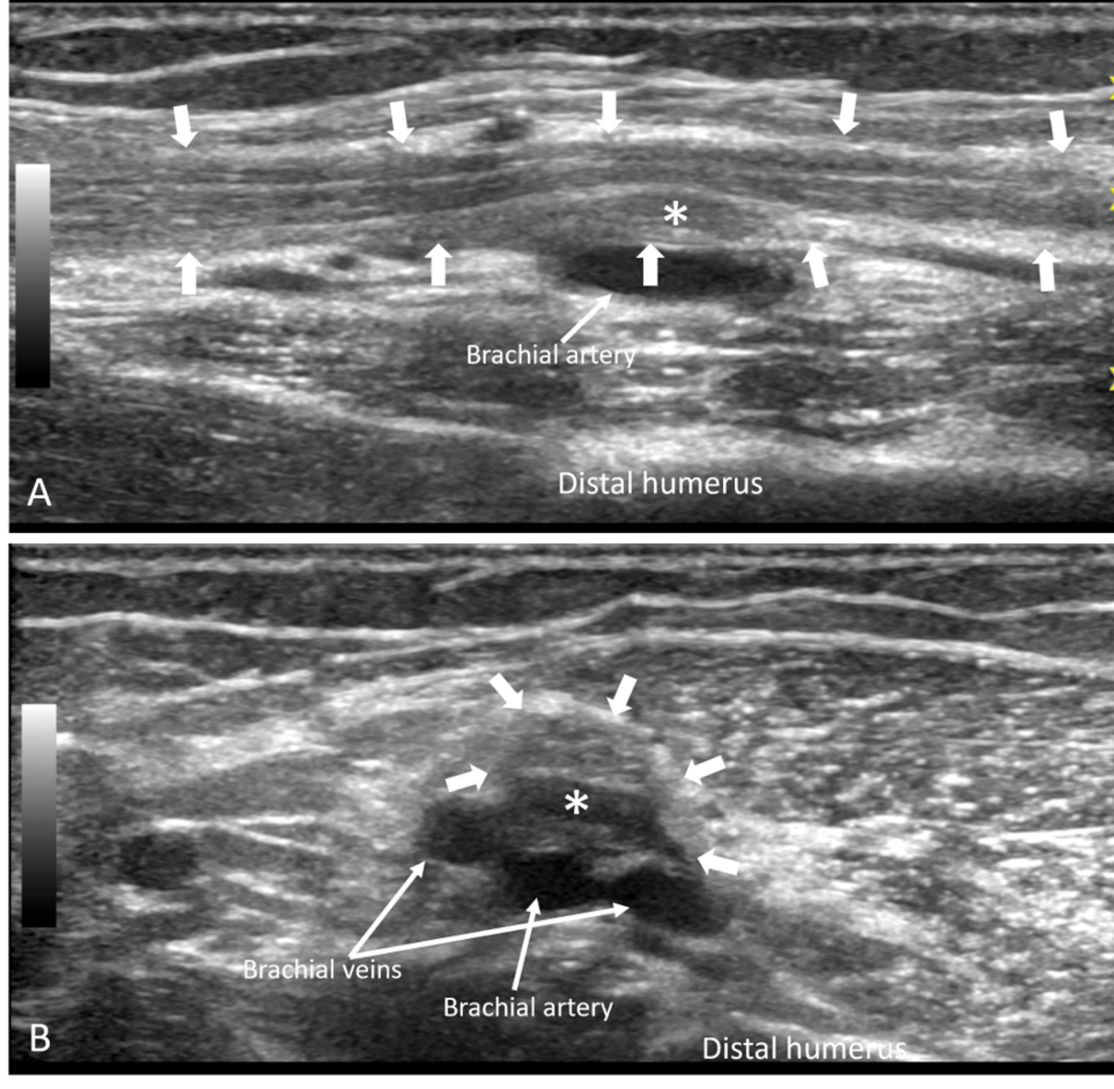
Follow-up median nerve ultrasound imaging in a 67-year-old woman with residual median neuropathy 4.5 months after traumatic PICC placement PICC: peripherally inserted central catheter Longitudinal (A) and transverse (B) grayscale US images demonstrate a marked interval decrease in size of the subepineural hematoma (*) encased within the median nerve epineurium (block arrows). The mass exerts much less mass effect on the fascicles, which are clearly distinguished
